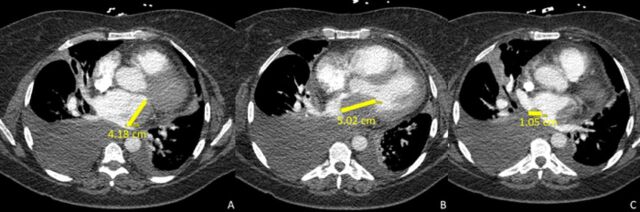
(A, B, C) Computed tomography shows 3 distinct left atrial masses adherent to the left atrium (measuring 4.18 cm, 5.02 cm, and 1.05 cm) with right-sided pleural effusion.
